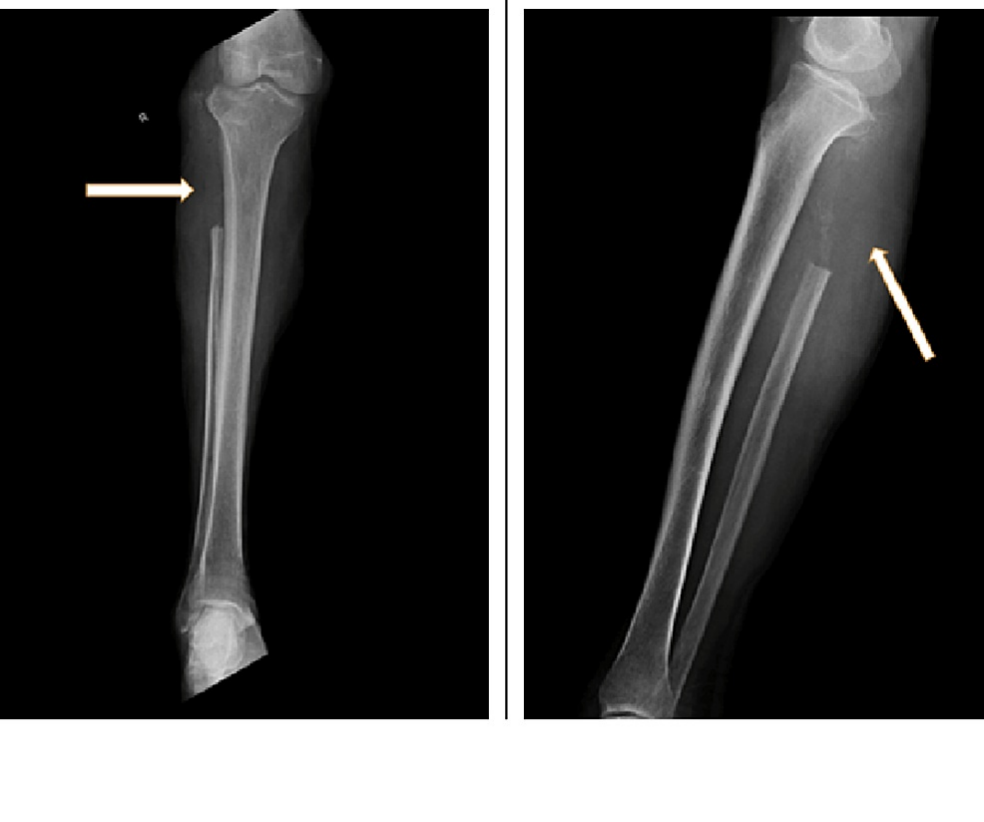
Twelve months follow-up X-ray showing no evidence of local recurrence.
